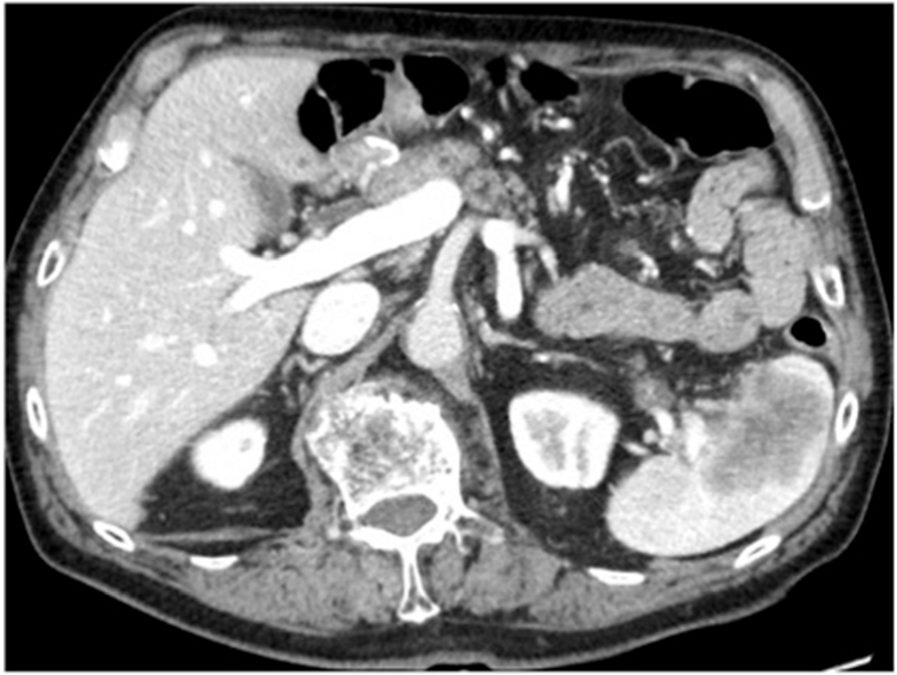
A hypodense lesion (diameter 5 cm) was identified on enhanced abdominal CT 23 months post-first surgery. CT, computed tomography
